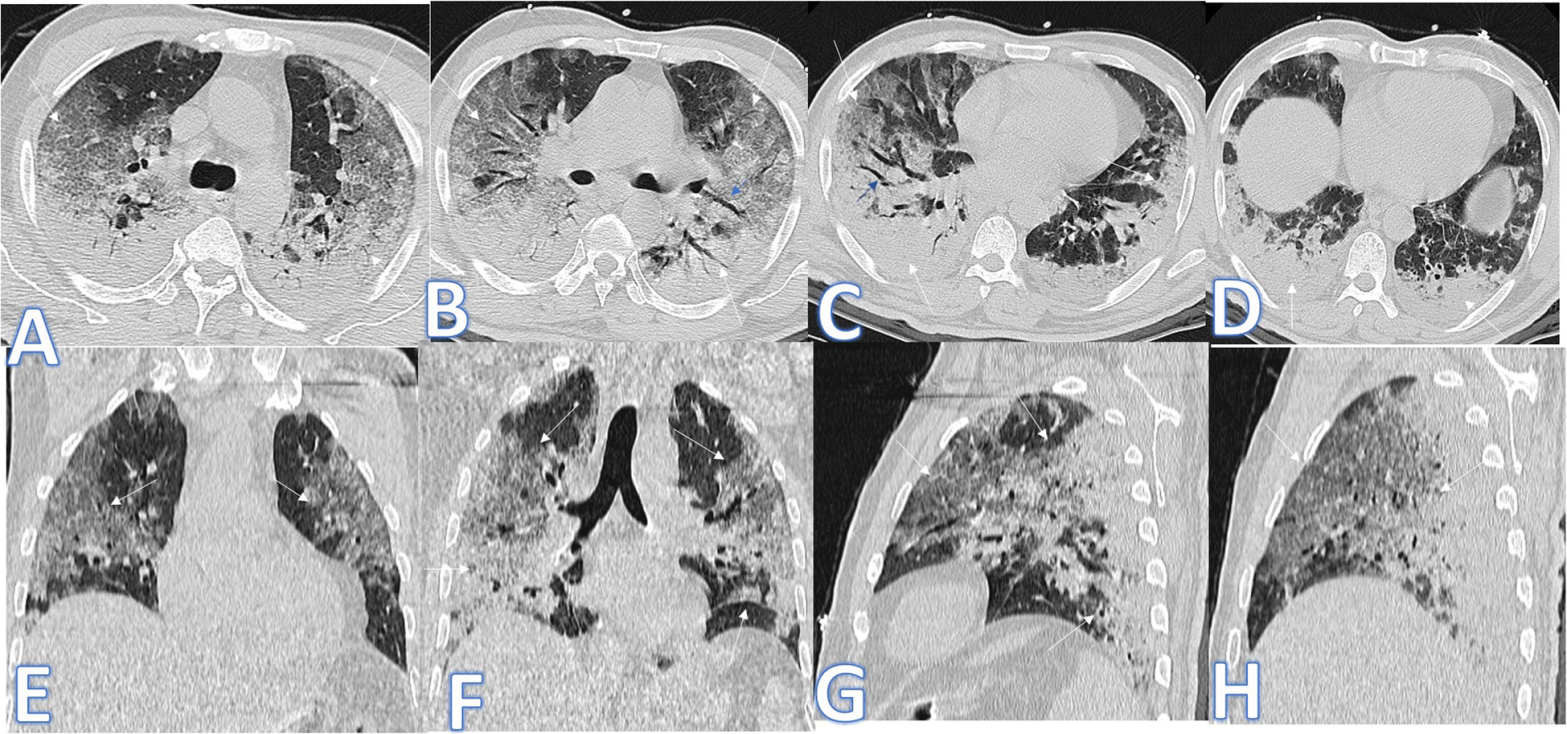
A 68-year-old male patient with septicemia, presented with fever and cough. CT showed bilateral basal dense consolidations on background of GGO in the anterior non-dependent portions of the lungs and crazy paving appearance (white arrows) with bronchial dilatation in the ground glass opacities (small blue arrows) with no pleural effusion, and the diagnosis was ARDS
